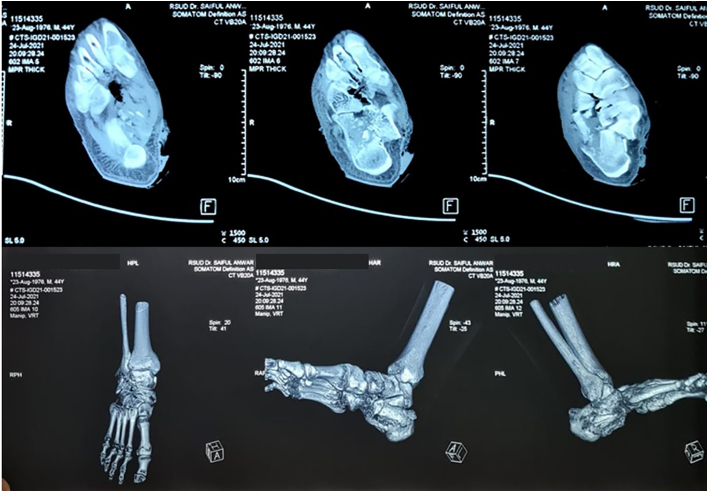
CT Scan of Calcaneal fracture pre-operative management.
